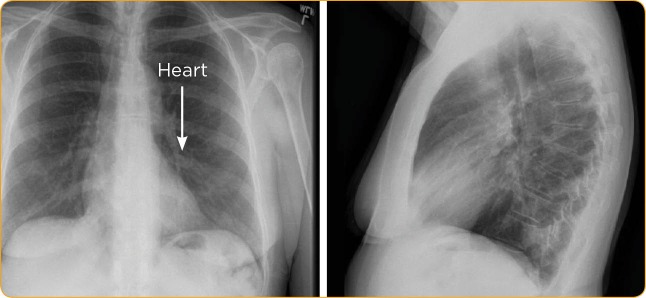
Posteroanterior (left) and lateral (always left-sided; right) views of chest x-ray of patient in Case Study 2.
